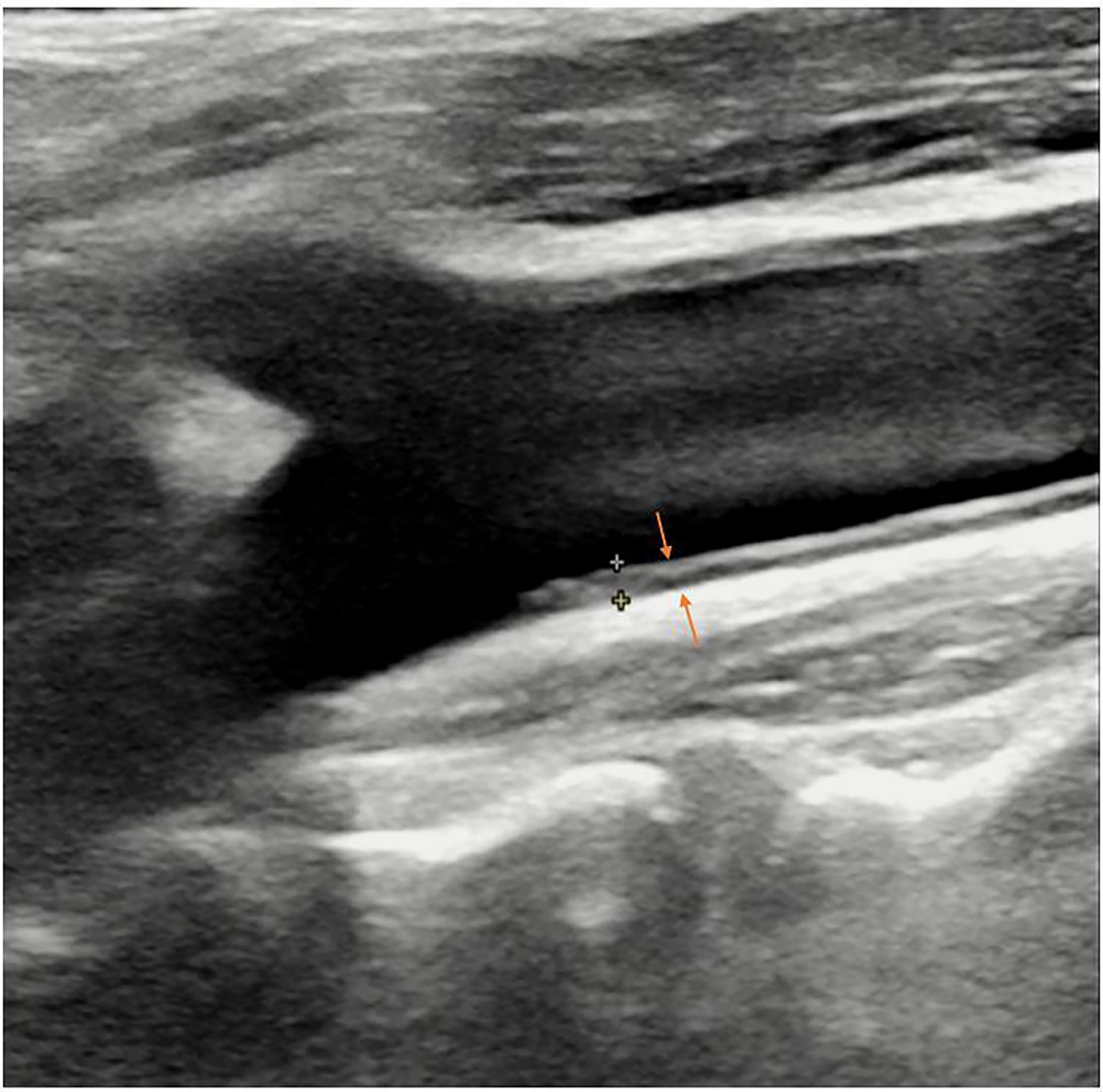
B-mode ultrasound image of carotid intima-media thickness (IMT) (arrows).
